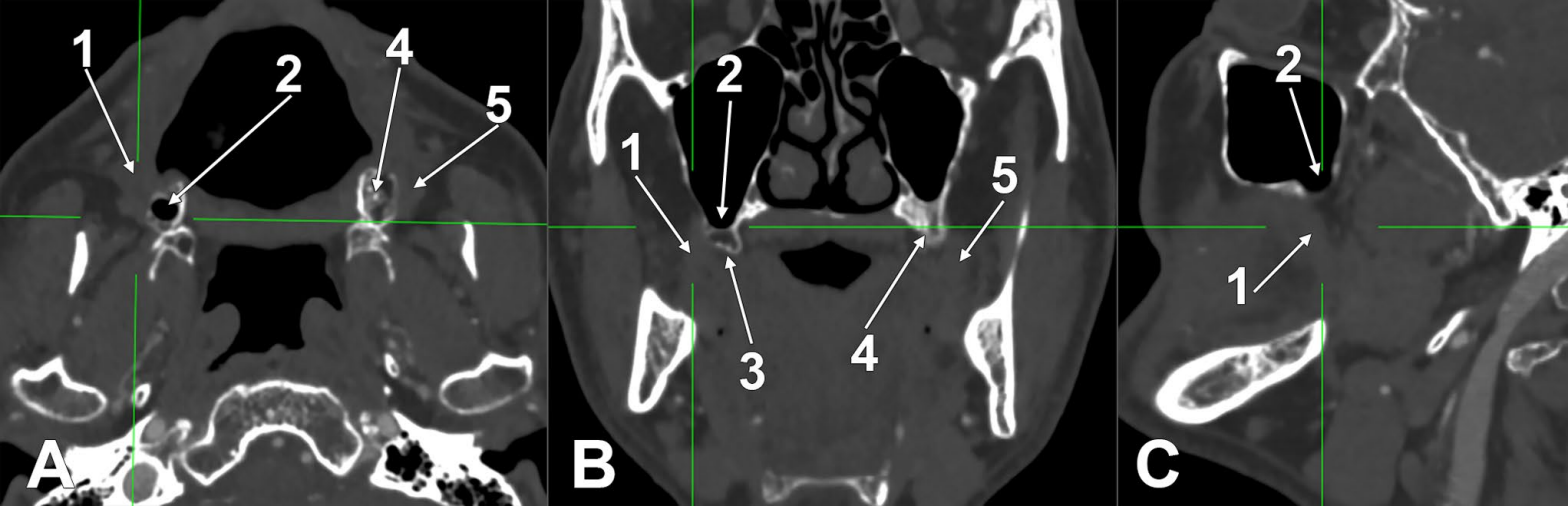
Residual alveolar tuberosity (AT) in corresponding CBCT slices. A. Axial slice, inferior view. B. Coronal slice, anterior view. C. Sagittal slice, medial view. (1) right buccinator muscle; (2) distal alveolar recess of the right antral floor, pneumatizes the right AT; (3) right AT; (4) left AT; (5) left buccinator muscle
